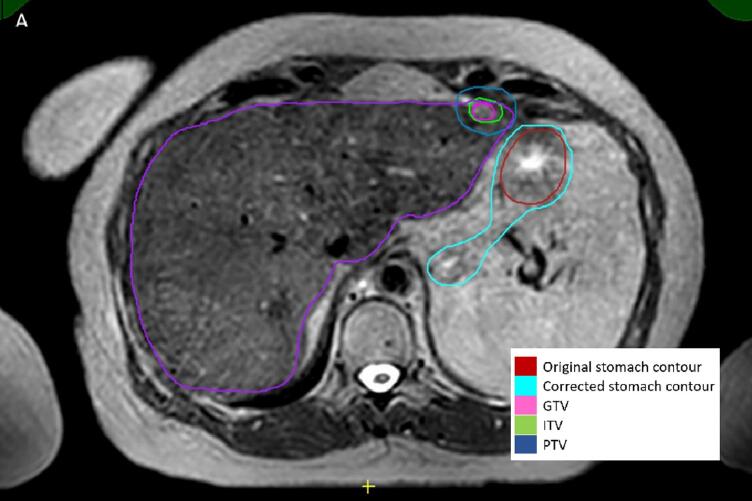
T2 navigated MRI scan of a patient with metastatic rectal adenocarcinoma with oligoprogressive segment 2 liver metastasis. Original stomach contour is denoted by dark red line. Pink, green and dark blue contours denote GTV, ITV and PTV. Turquoise line shows corrected stomach contour after peer review.
